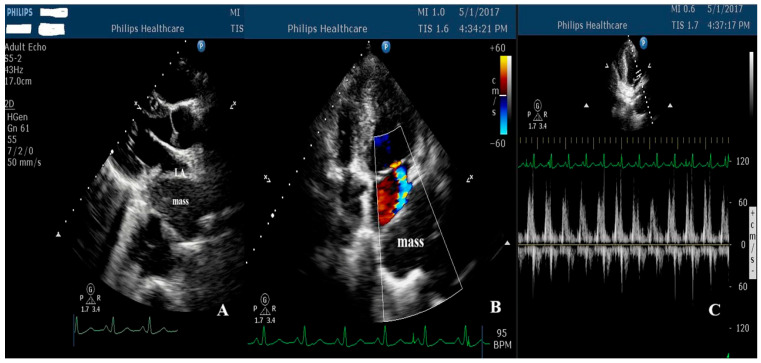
Transthoracic echocardiography showing hemodynamic consequences of an extracardiac mass. (A) Parasternal long-axis view showing the mass located posterior to the left atrium. (B) Apical four-chamber view with color Doppler imaging showing the mass adjacent to the left atrium, without evidence of internal vascular flow. (C) Pulsed-wave Doppler of the transmitral inflow demonstrating pronounced respiratory variations in flow velocities, reflecting functional compression of the left atrium.
